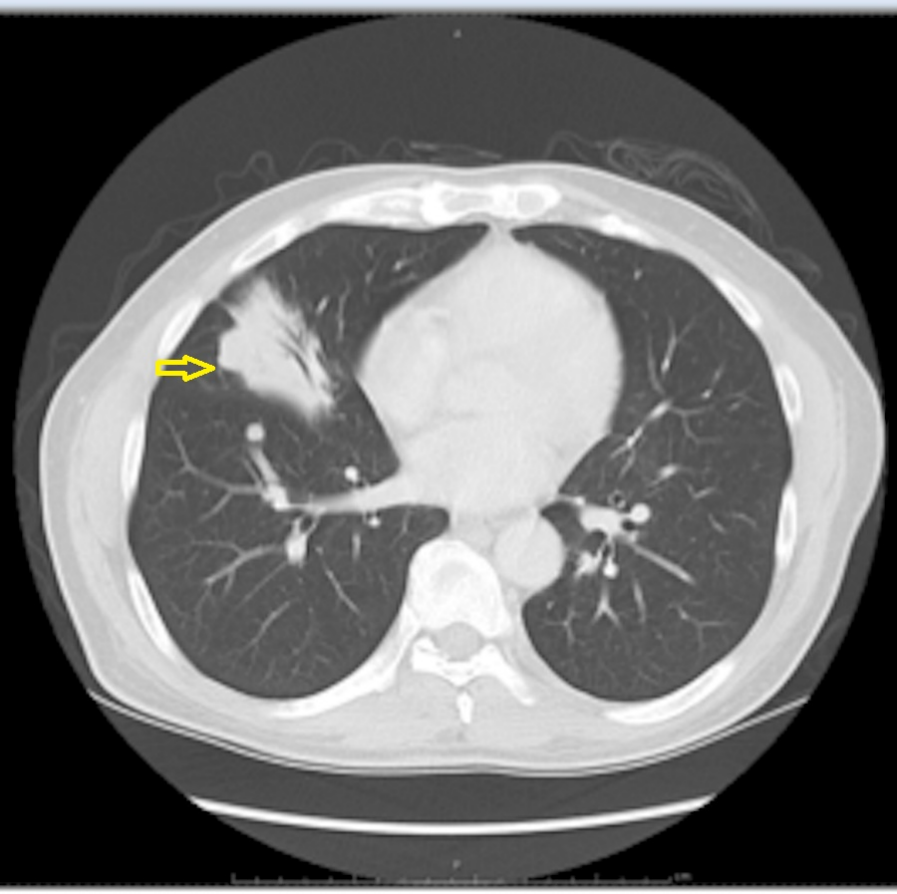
Computerized tomography (CT) of the chest showing right middle lobe opacity (arrow).
